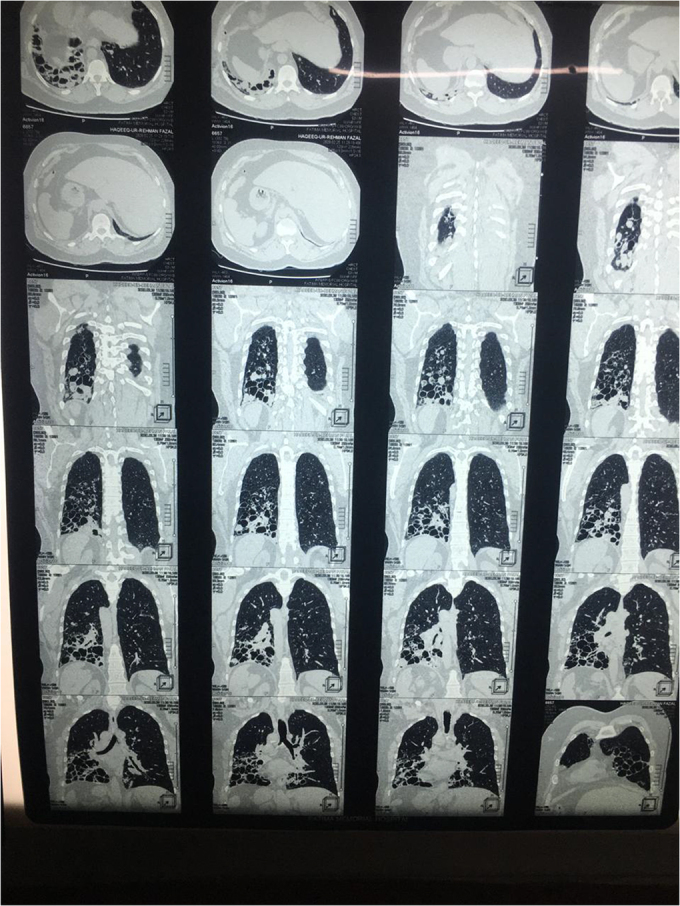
HRCT chest showed a right-sided heart along with cystic bronchiectatic changes in both the right and left lower lobes of the lung.
